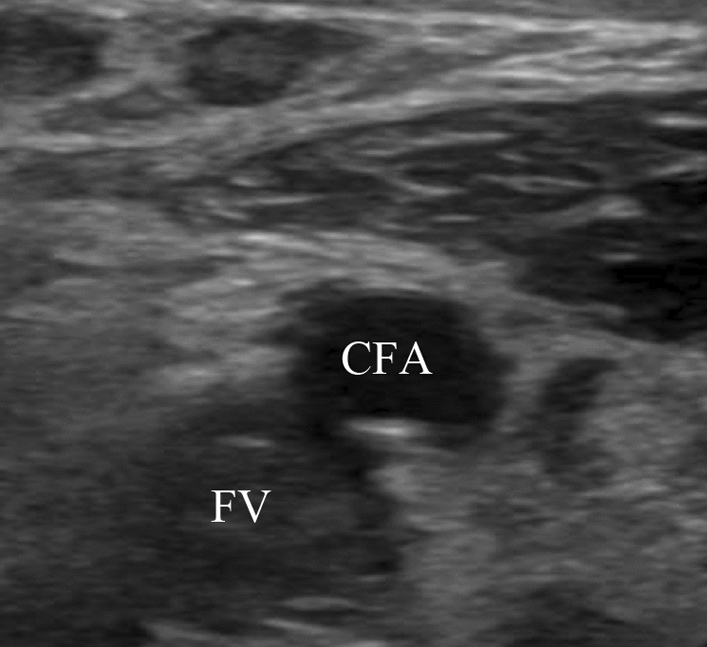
Ultrasound showing thrombus can be seen in left femoral vein
